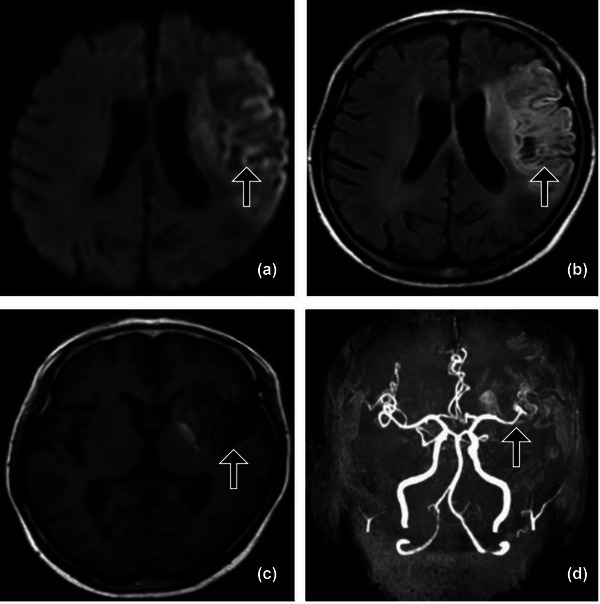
ICAS patient. A 73‐year‐old male was admitted to the hospital 10 h after stroke. A: DWI, B: T2WI‐FLAIR, C: T1WI, D: MRA. Massive infarct in the left cerebral hemisphere, left MCA, and bilateral PCA atherosclerotic stenosis.
